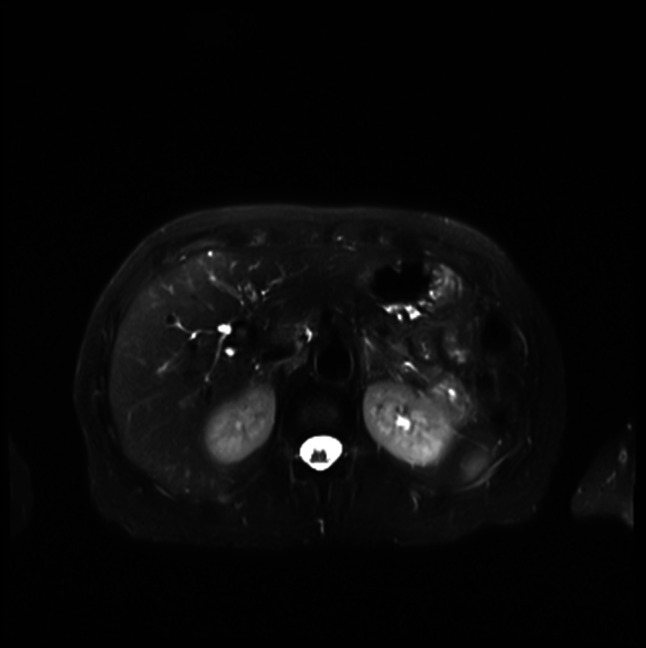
MRI of the abdomen showing cystic lesion at the uncinate process, likely a side branch IPMN. MRI, magnetic resonance imaging.
